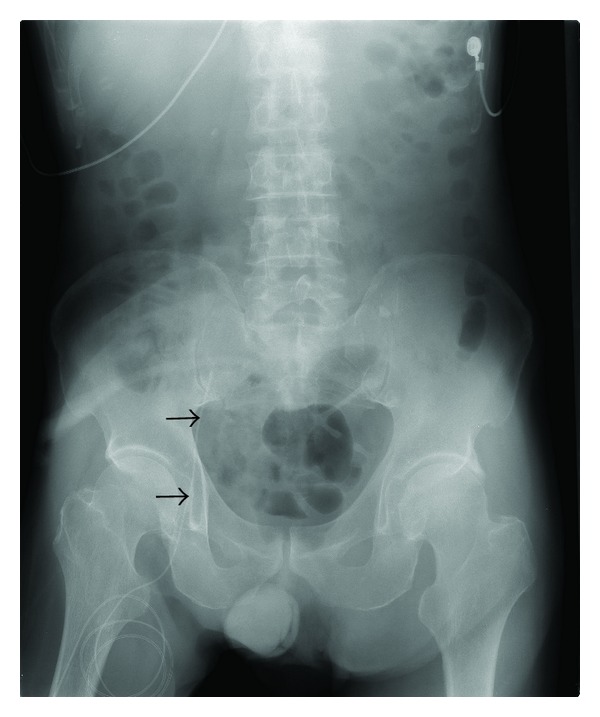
A plain radiograph confirms the position of the catheter.
